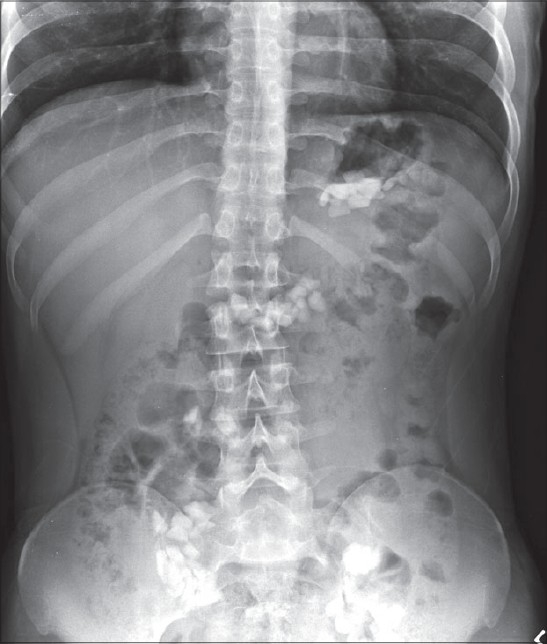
Plain X-ray of the abdomen showing multiple radio-opaque shadows in the colon
